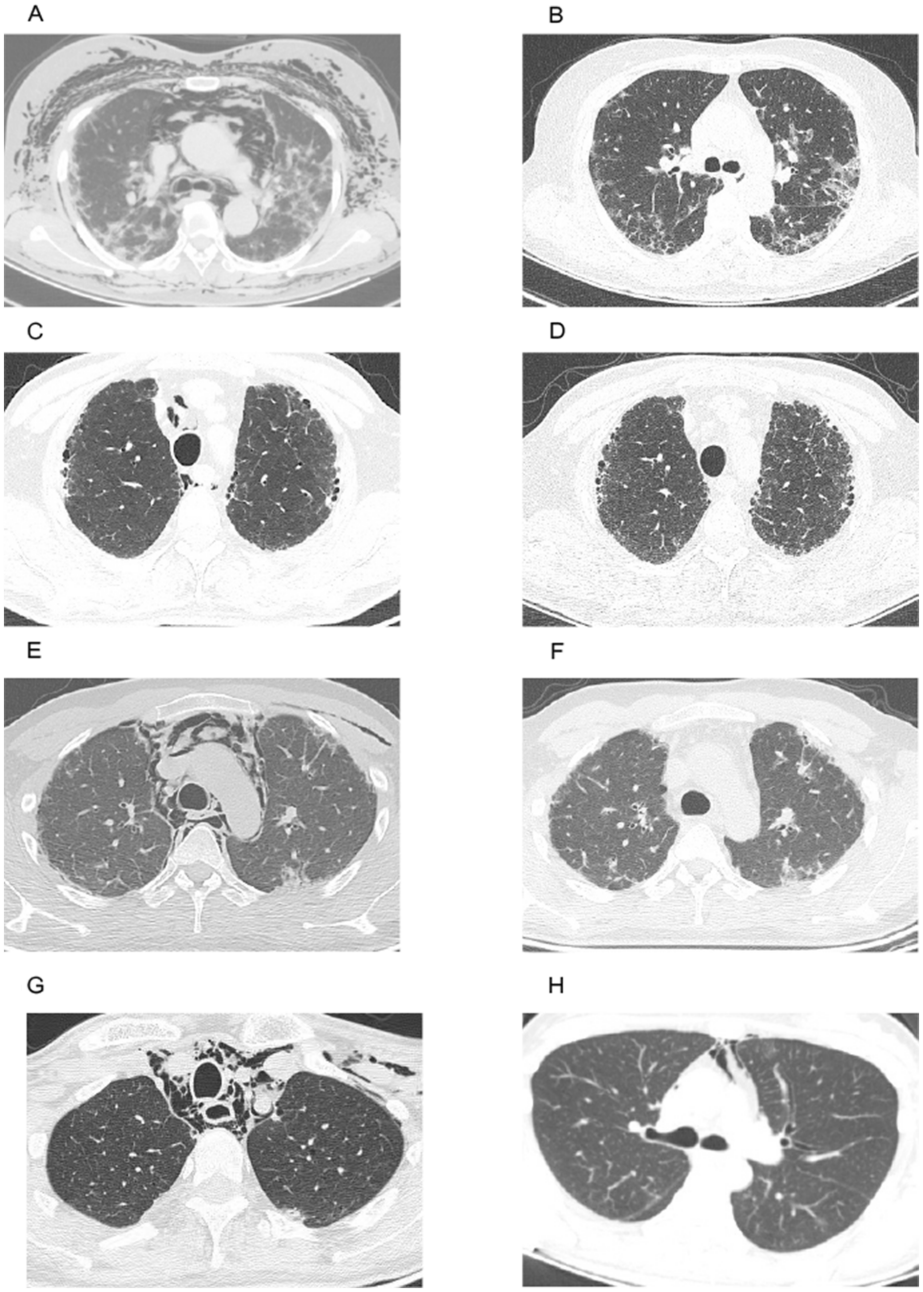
(A) Chest CT scan revealed bibasilar consolidations indicating interstitial lung disease, extensive pneumomediastinum, and subcutaneous emphysema. (B) Chest CT scan after combination therapy with tofacitinib, pneumomediastinum, and subcutaneous emphysema resolved. (C) Chest CT scan revealed ground-glass opacities and pneumomediastinum. (D) Chest CT scan after combination therapy with tofacitinib, pneumomediastinum resolved. (E) Chest CT scan revealed multiple bilateral peripheral and subpleural areas of consolidation. (F) Chest CT scan after combination therapy with tofacitinib, pneumomediastinum, and subcutaneous emphysema resolved. (G) Chest CT scan revealed bibasilar consolidations and extensive pneumomediastinum and subcutaneous emphysema. (H) Chest CT scan revealed pneumomediastinum.
